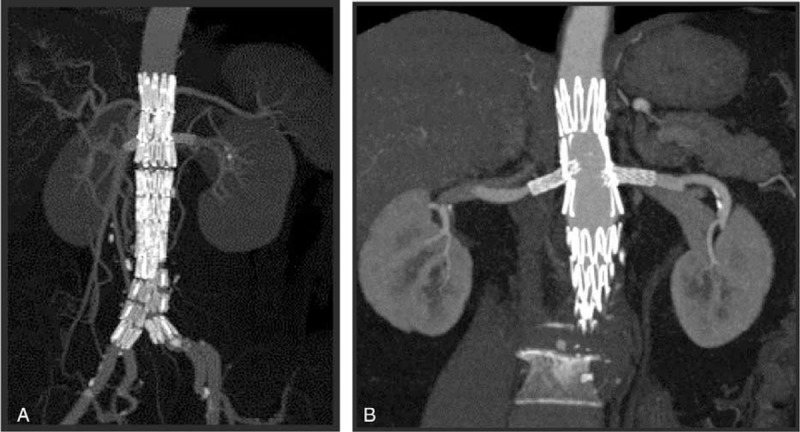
(A, B) Two computed tomography angiography images showing patency of 2 renal arteries without endoleaks and endograft migration at 1 year after the operation.
